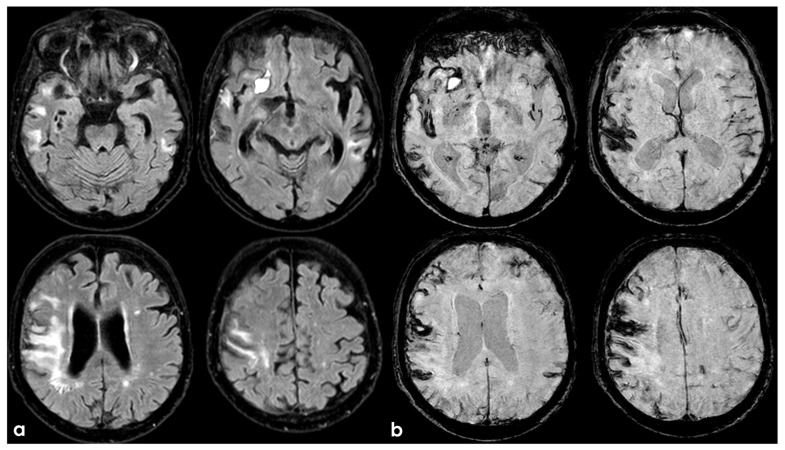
Brain MRI showing axial FLAIR (panel (a)) and axial Susceptibility Weighted Imaging (SWI) minimum intensity projection (panel (b)) slices. The new hemorrhage was hyperintense on both sequences, and the assed cortical superficial siderosis was well identifiable in comparison with previous MRI.
